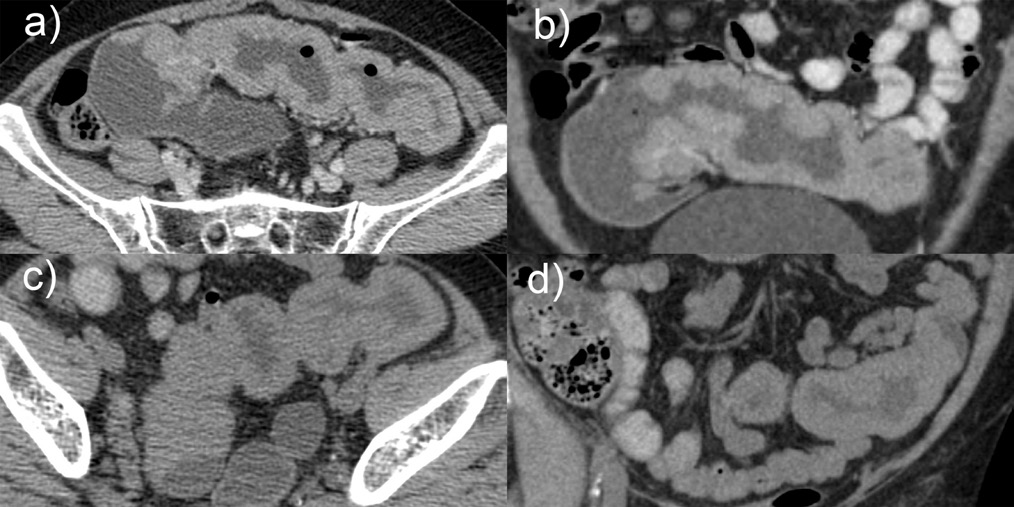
CT abdomen demonstrating segmental wall thickening of homogeneous density in the sigmoid colon. Displayed are post-i.v. and oral contrast axial (a) and coronal (b) plane images, compared with non-contrast axial (c) and coronal (d) plane images
